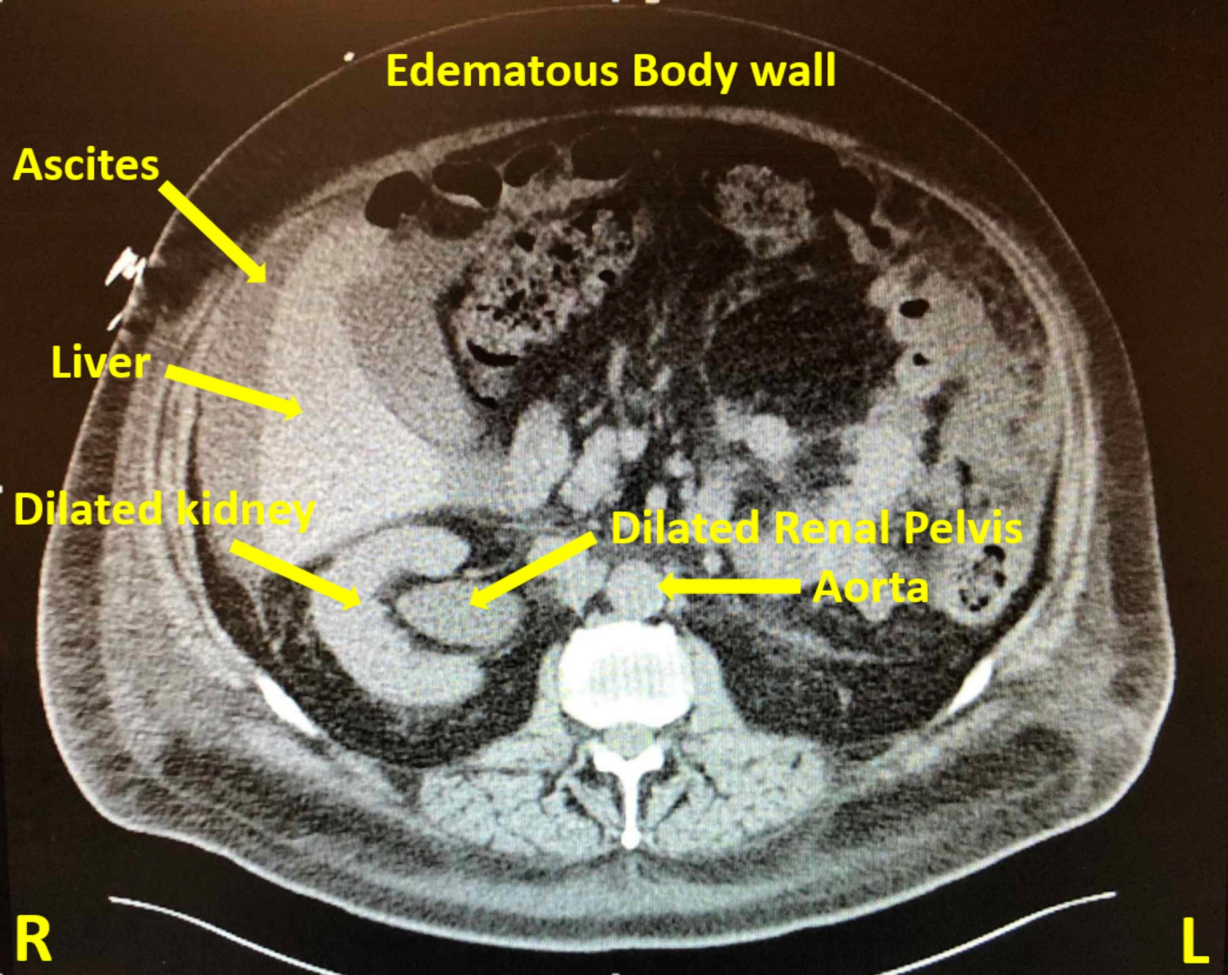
Computed tomography (CT) of the abdomen/pelvis with oral contrast The scan revealed moderate right hydro-uretero-nephrosis extending all the way to the uretero-vesicular junction; wall thickening along the superior and posterior margin of the bladder; moderate-large right pleural effusion; moderate abdominal and pelvic ascites; generalized anasarca of the soft tissues.
